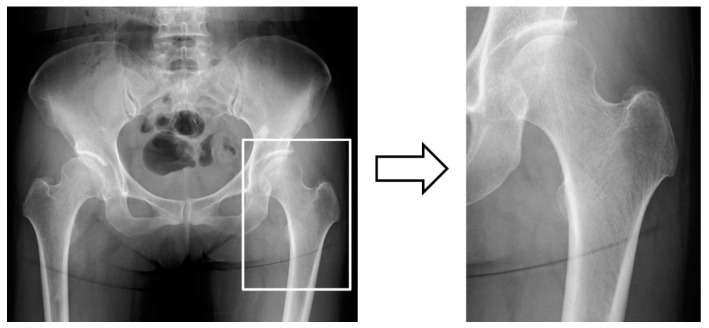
Crop method as data preprocessing. The manually cropped area perfectly imitated the osteoporosis identification range obtained using the dual-energy X-ray absorptiometry (DXA) method.
